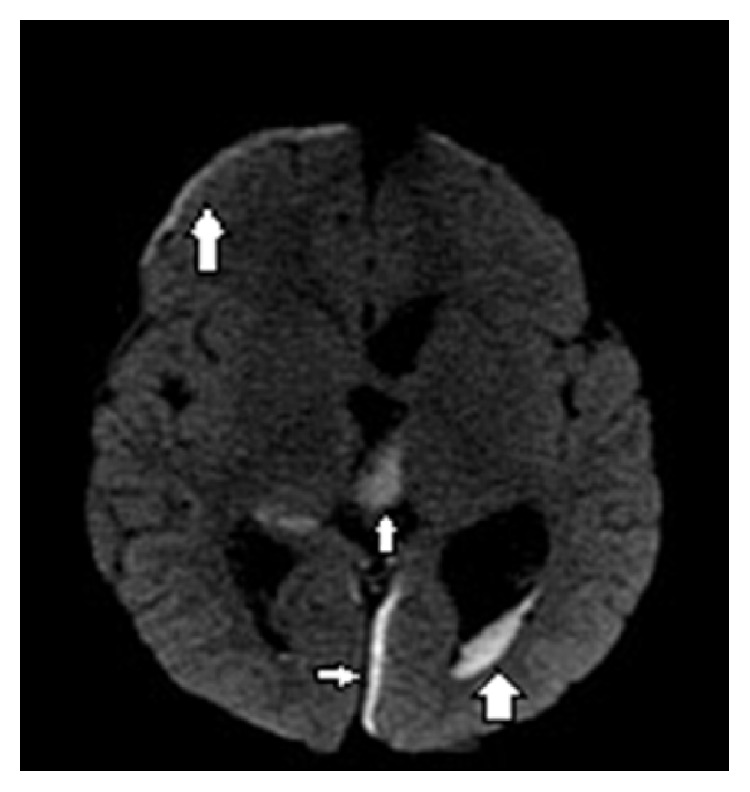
Brain MRI on day 5 for meningitis evaluation. Axial diffusion weighted MR image of the brain shows restricted diffusion over the right frontal lobe and left medial occipital lobe and in the ventricles suggesting subdural empyemas and intraventricular abscesses (arrows).
